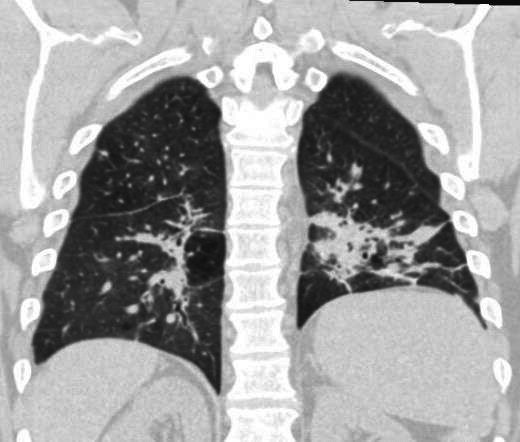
Coronal CT chest with significant improving consolidations and nodular infiltrates in both lungs.
